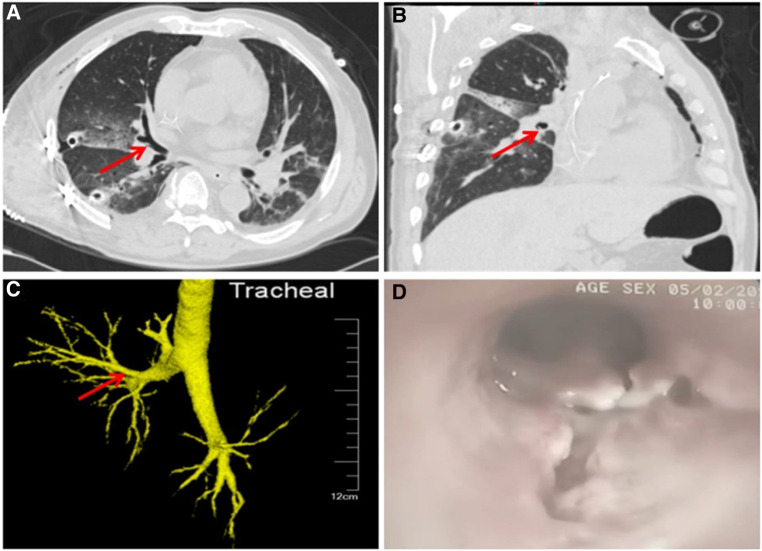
Axial (A) and sagittal (B) view of CT shows the laceration of the bronchial wall in the middle lobe of right lung. The red arrow indicates the location of the laceration. CT 3D reconstruction (C) shows that the bronchi are continuous and intact, while the laceration is clearly demonstrated in bronchoscopy (D).
